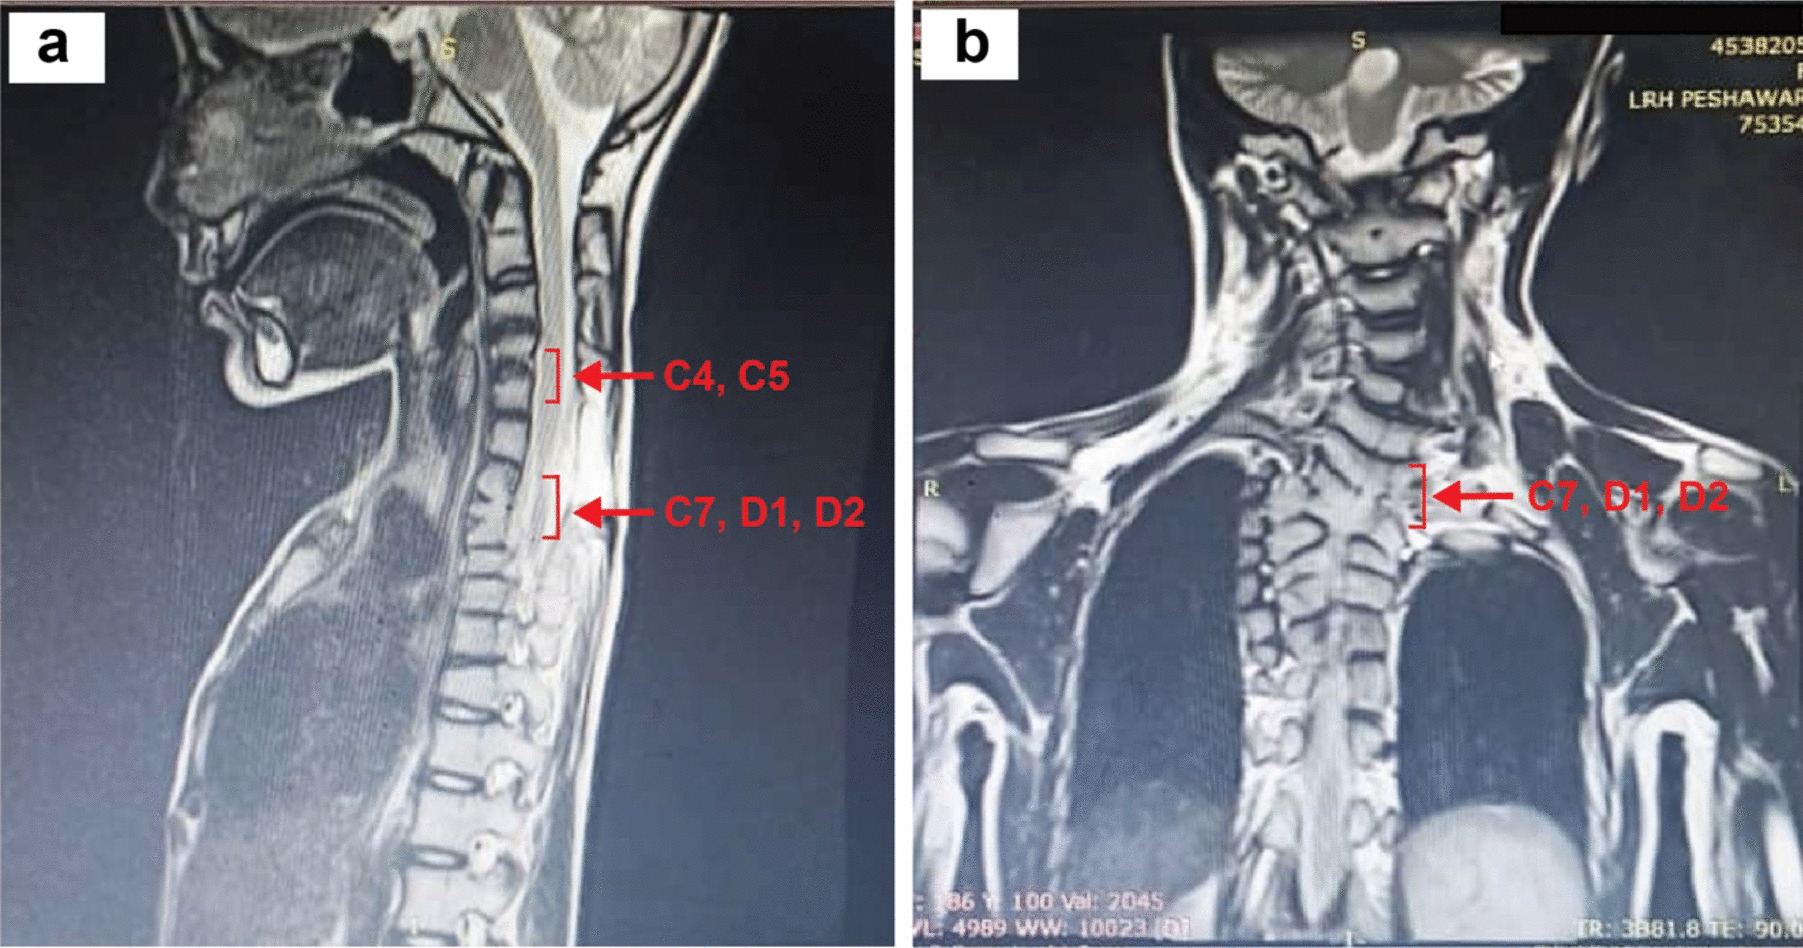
T2-weighted imaging of cervical spine of a 12-year-old female patient born with KFS (Type III). (A) Saggital view indicating hemivertebrae at the C4–C5 levels and hemivertebrae with fusion at the C7, D1, and D2 levels. (B) Coronal view showing hemivertebrae with fusion at the C7, D1, and D2 levels (red arrows indicating fused vertebrae)
